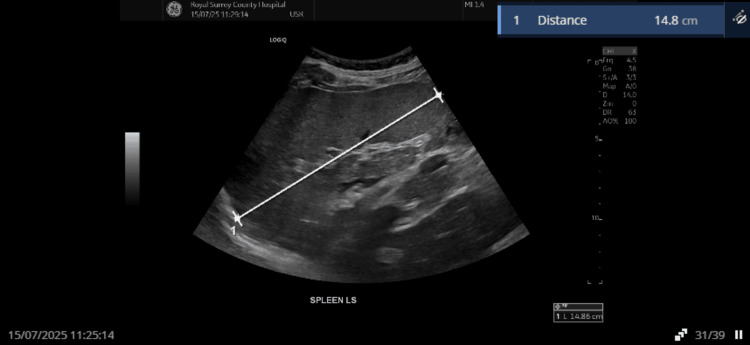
Splenomegaly detected on the next-day abdominal ultrasound scan Abdominal ultrasound demonstrating splenomegaly (14.8 cm) initially raising concern for infarct, haematoma, or other pathology.
